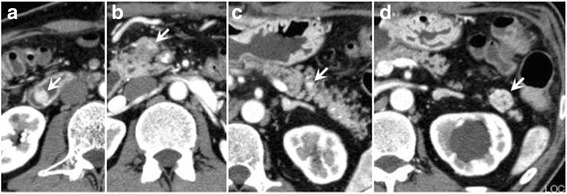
Enhanced computed tomography showing multiple highly vascular tumors within the pancreas and the duodenum; a well demarcated duodenal tumor protruding into the lumen (a), a tumor at the head (b), body (c), and tail (d) of the pancreas
